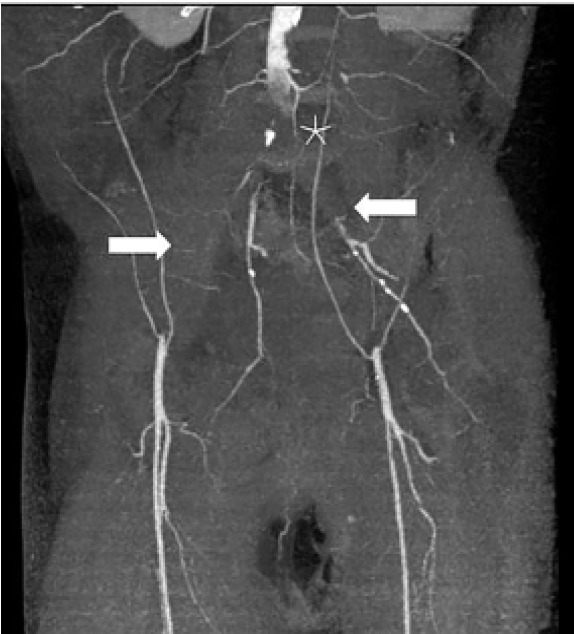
Computed tomographic angiography of abdomen and lower extremities with extensive thrombosis of distal abdominal aorta (asterisk) and occlusion of bilateral iliac arteries (arrows).
